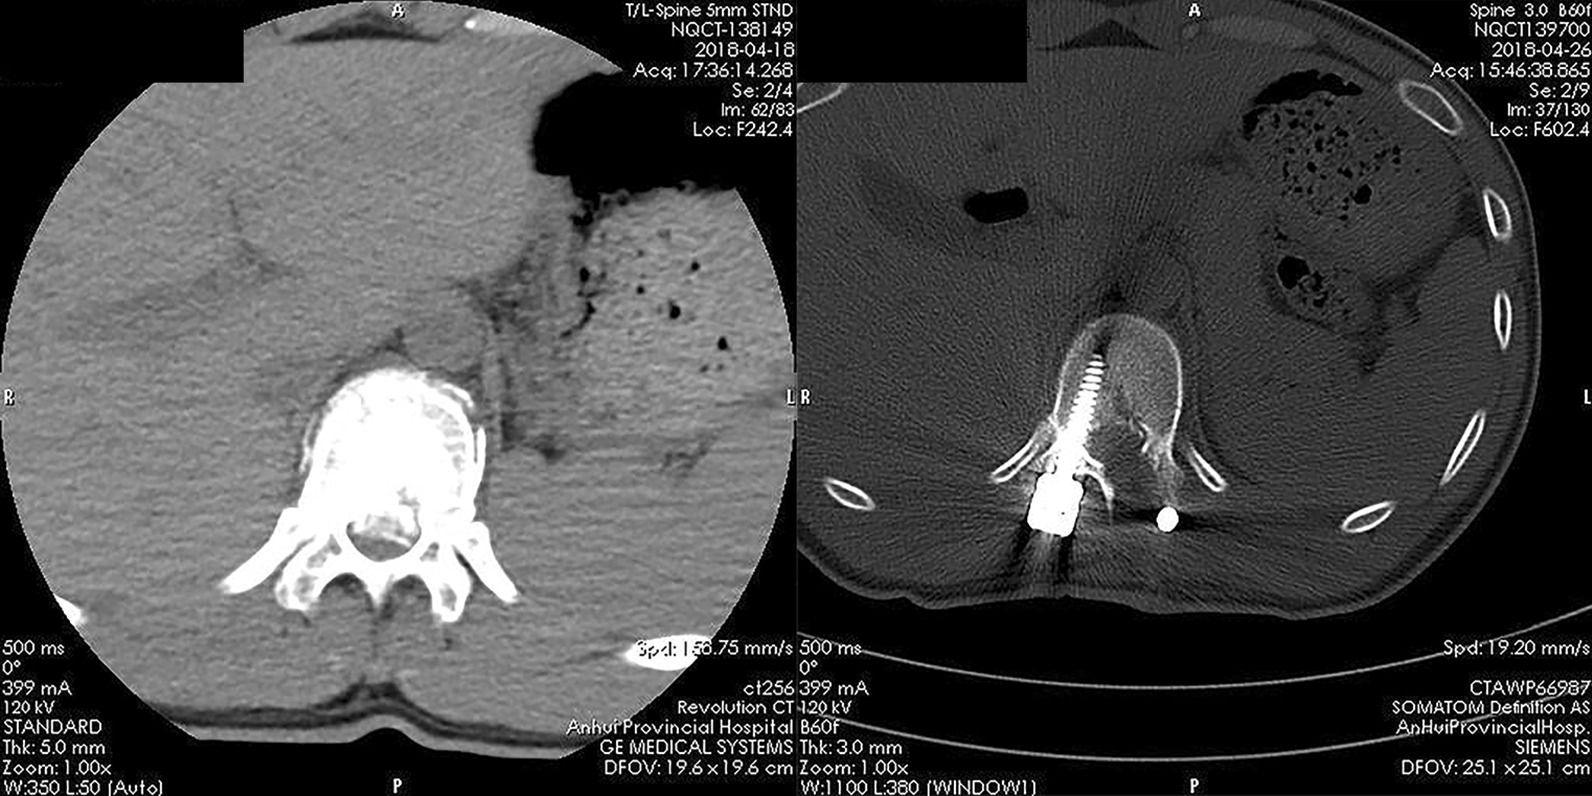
Computed tomography demonstrated that the bone fragment that had herniated into the spinal canal (A) was successfully reduced (B)
